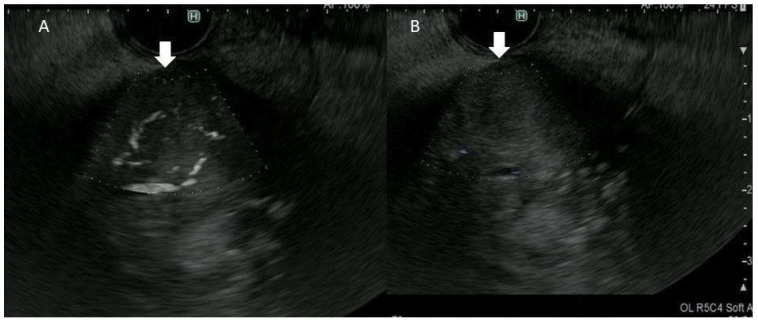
The difference between detective flow imaging (DFI) and e-FLOW endoscopic ultrasonography for vessel detection in malignant gallbladder lesion. (A) DFI-endoscopic ultrasonography: the vessels were depicted in the gallbladder lesion (arrow). (B) e-FLOW endoscopic ultrasonography: the vessels were not depicted in gallbladder lesion (arrow).
